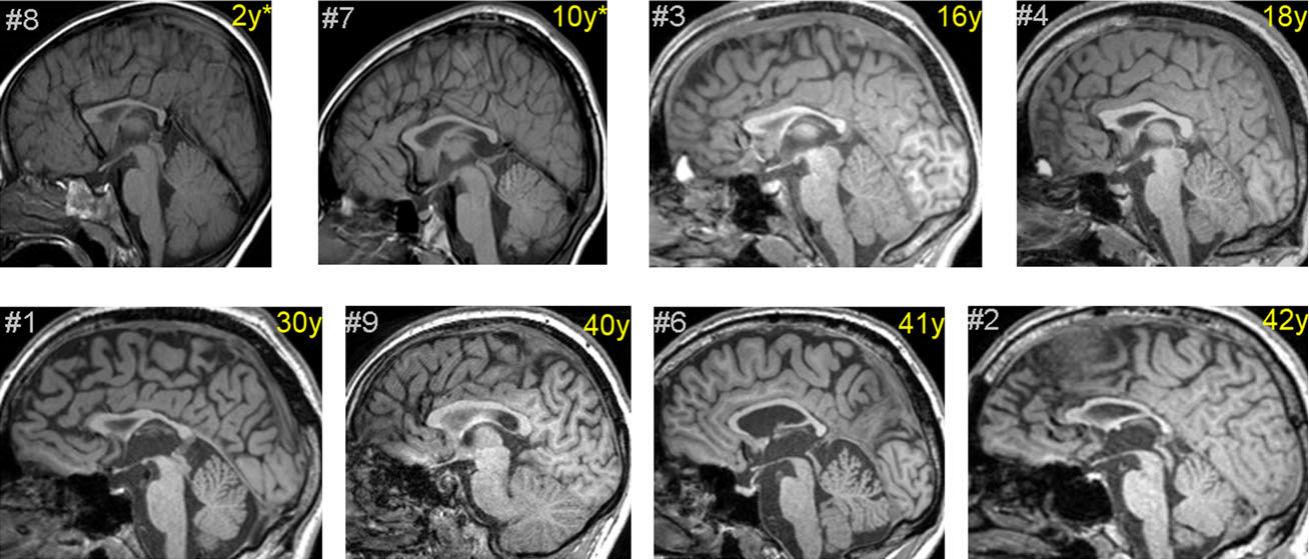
Magnetic resonance imaging for males with *ZDHHC9* mutations. T1-weighted MRI (midsagittal slice) for eight males with *ZDHHC9* mutations. Scans are ordered by chronological age at the time of MRI image acquisition. *Clinically acquired scans. #Case number in Table S1.
